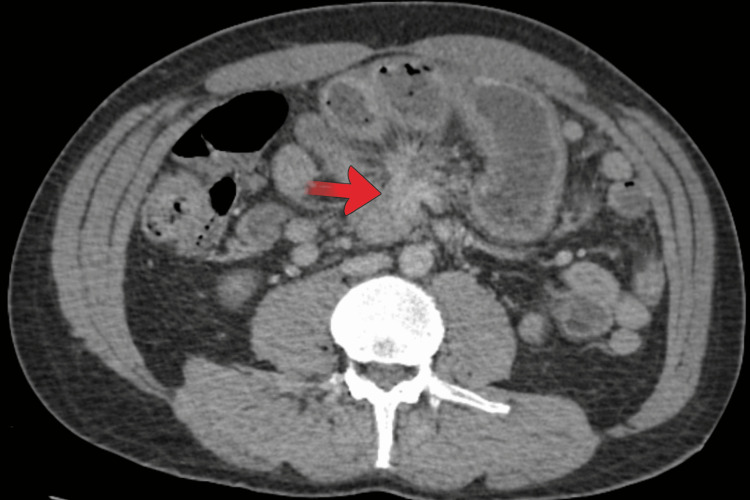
Contrast-enhanced CT of the abdomen and pelvis demonstrating a mesenteric mass (red arrow) at the transition point of small bowel obstruction. Converging mesenteric vessels and surrounding mesenteric stranding are present, findings characteristic of the desmoplastic reaction associated with small bowel neuroendocrine tumors. Adjacent dilated small bowel loops are visible, consistent with a closed-loop obstruction and impending bowel ischemia.
